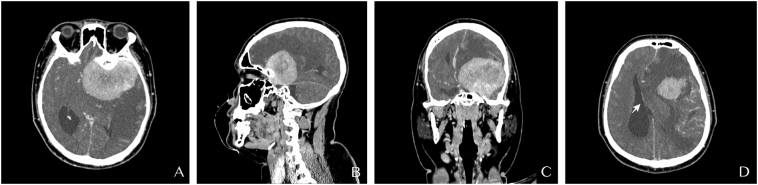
CT Scan with contrast showing extraaxial mass on the left sphenoid region, axial (A), sagittal (B), and coronal (C), which causes more than 2 cm of midline shift (arrow) and extensive perifocal edema (D).
